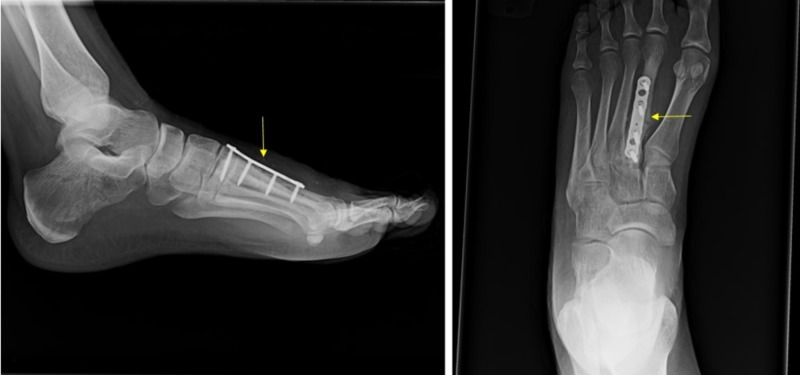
X-rays two weeks post-revision open reduction and internal fixation with plate and screws.
